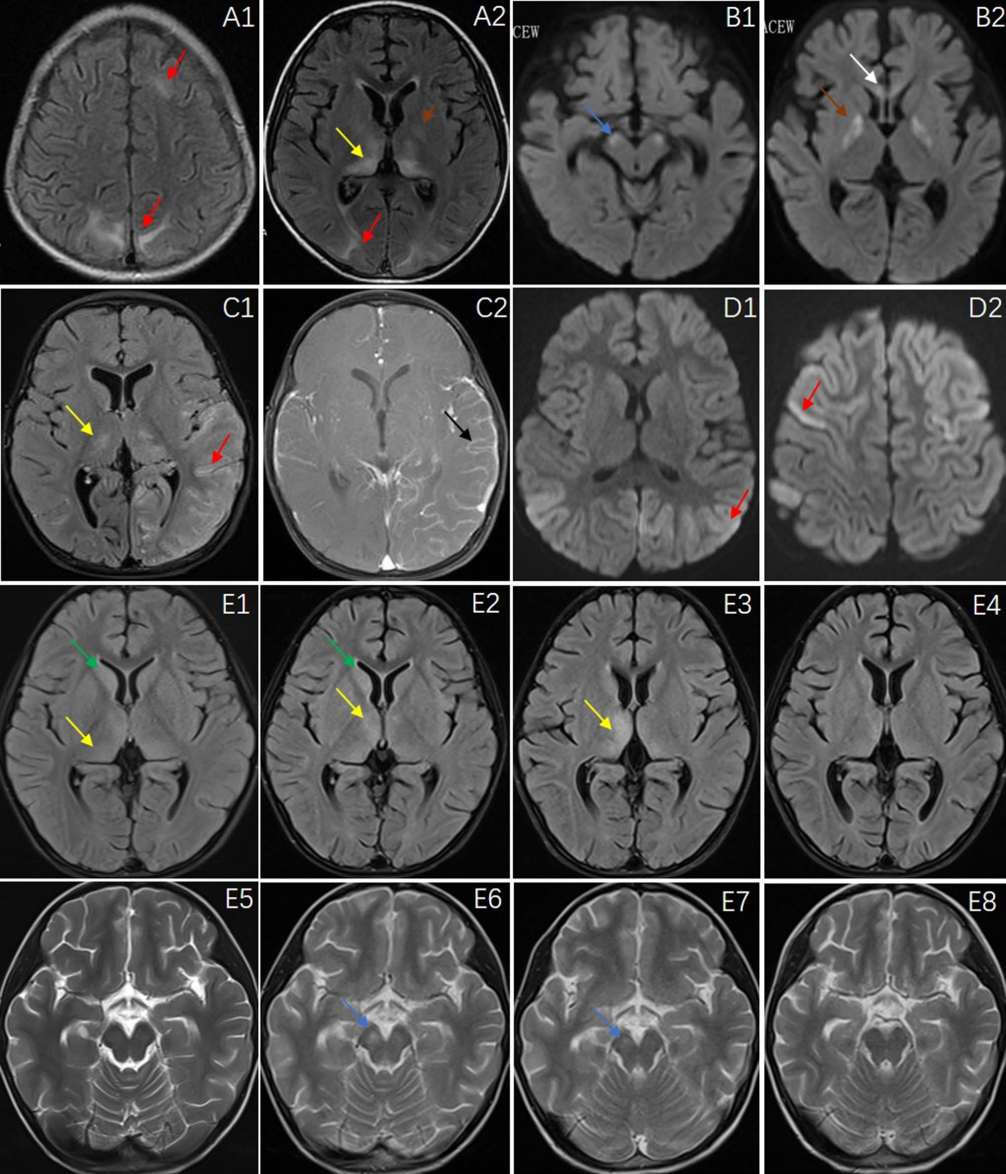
Brain MRI features of patients N.3, N.5, N.6, N.8 and N.10. Patient N.3, a 6-year-old boy, had a clinical-radiological presentation of Morvan syndrome including multifocal lesions in the occipital and parietal cortex and thalamus (A1, A2). Patient N.5, a 5-month-old boy, presented with encephalopathy and bilateral MRI abnormalities in the globus pallidus, corpus callosum and cerebral peduncle (B1, B2). Patient N.6, a 2-year-old girl, had a clinical-radiological presentation of autoimmune encephalitis, including cortical lesions in the frontal, parietal, occipital and temporal lobes and thalamus and cortical lesions showing enhancement with gadolinium administration (C1, C2). Patient N.8, a 5-year-old girl, presented with two episodes of fever and seizures within a week. Brain MRI showed extensive cortical lesions in the frontal, parietal, occipital and temporal lobes (D1, D2). Patient N.10, a 6-year-old boy, presented with fever, ataxia, slow responses. Brain MRI (E1–E8) showed a series of changes including lesions in the thalamus, caudate nucleus and cerebral peduncle (E1/E5, E2/E6, E3/E7, E4/E8, representing D4, D16, D30, and D50 after onset, respectively). Fluid-attenuated inversion recovery sequences were used in (A1, A2, C1, E1–E4, and C2, which show enhancement with gadolinium administration. T2 sequences were used in (E5–E8). Diffusion weighted imaging was used in (B1, B2, D1 and D2). Red arrows represent multiple cortical lesions, yellow arrows: thalamus, blue arrows: cerebral peduncle, green arrows: caudate nucleus, brown arrows: globus pallidus, white arrow: corpus callosum, black arrow: meningeal enhancement.
